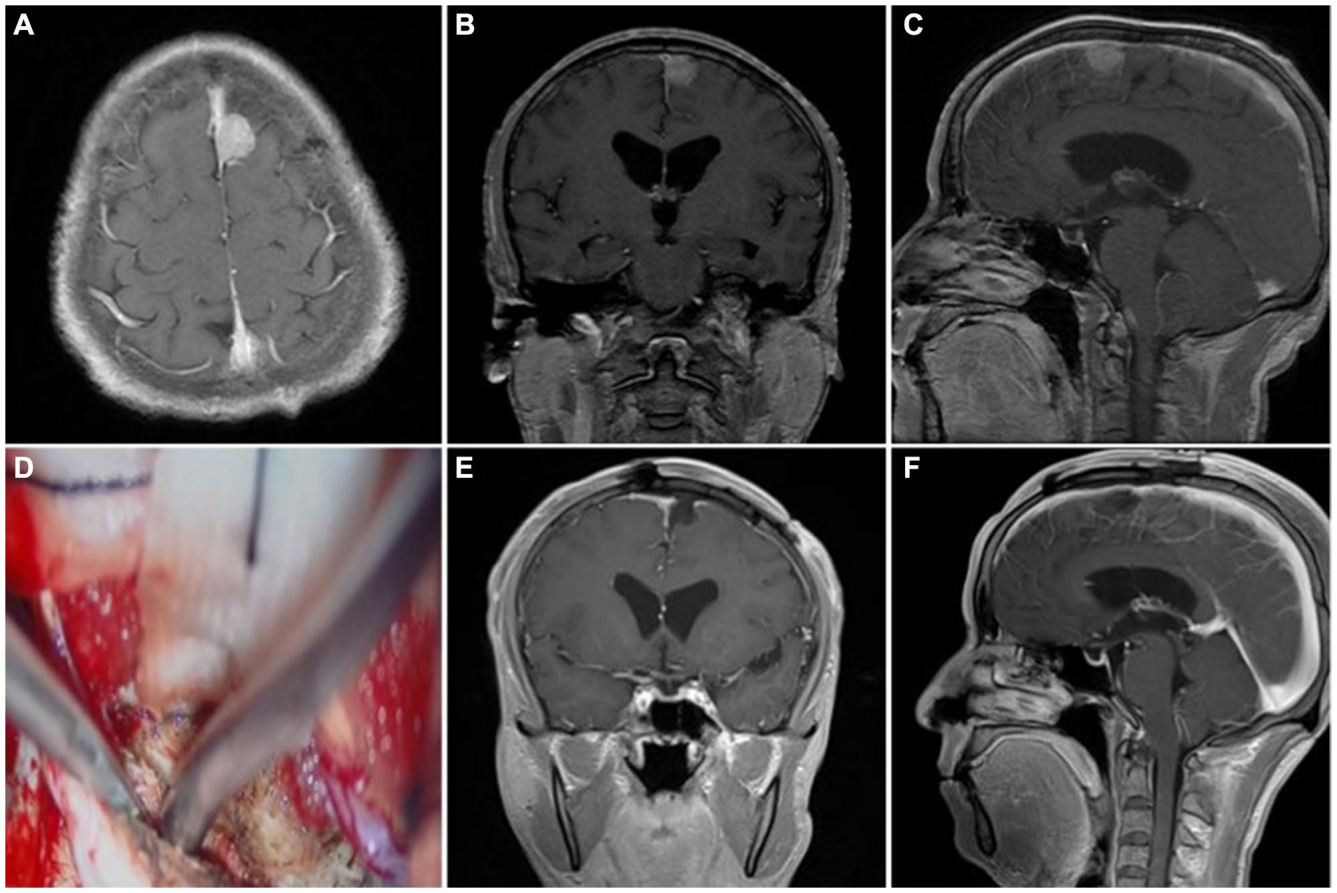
Representative case 1, type I (patent sinus). (A–C) Preoperative enhanced MRI images. (D) Intraoperative resection of the outer layer of the sinus wall. (E,F) Postoperative enhanced MRI images.
